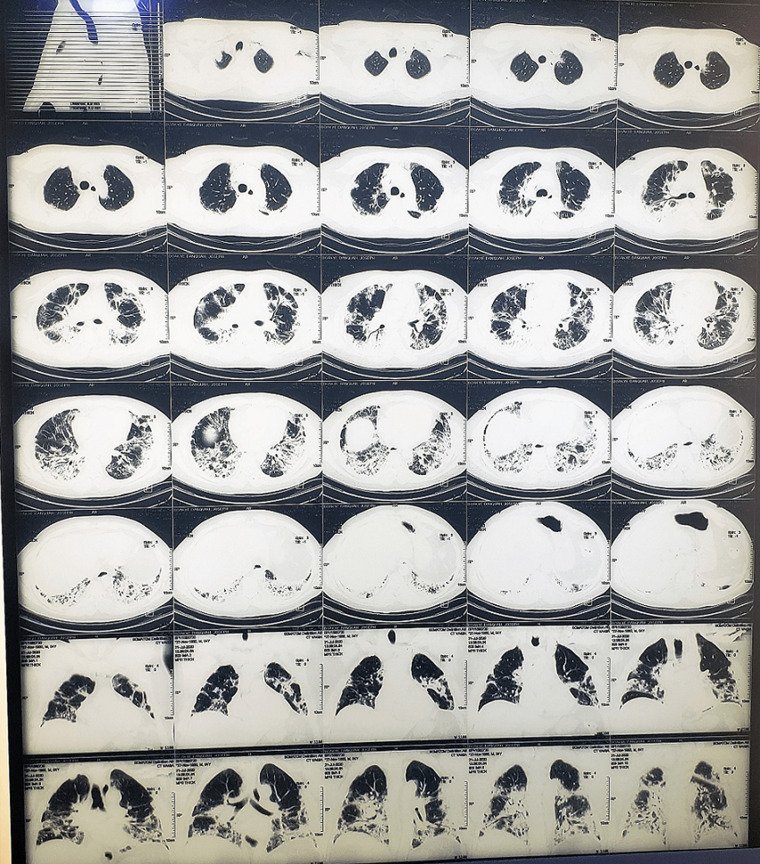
computed tomography pulmonary angiogram on day 7
